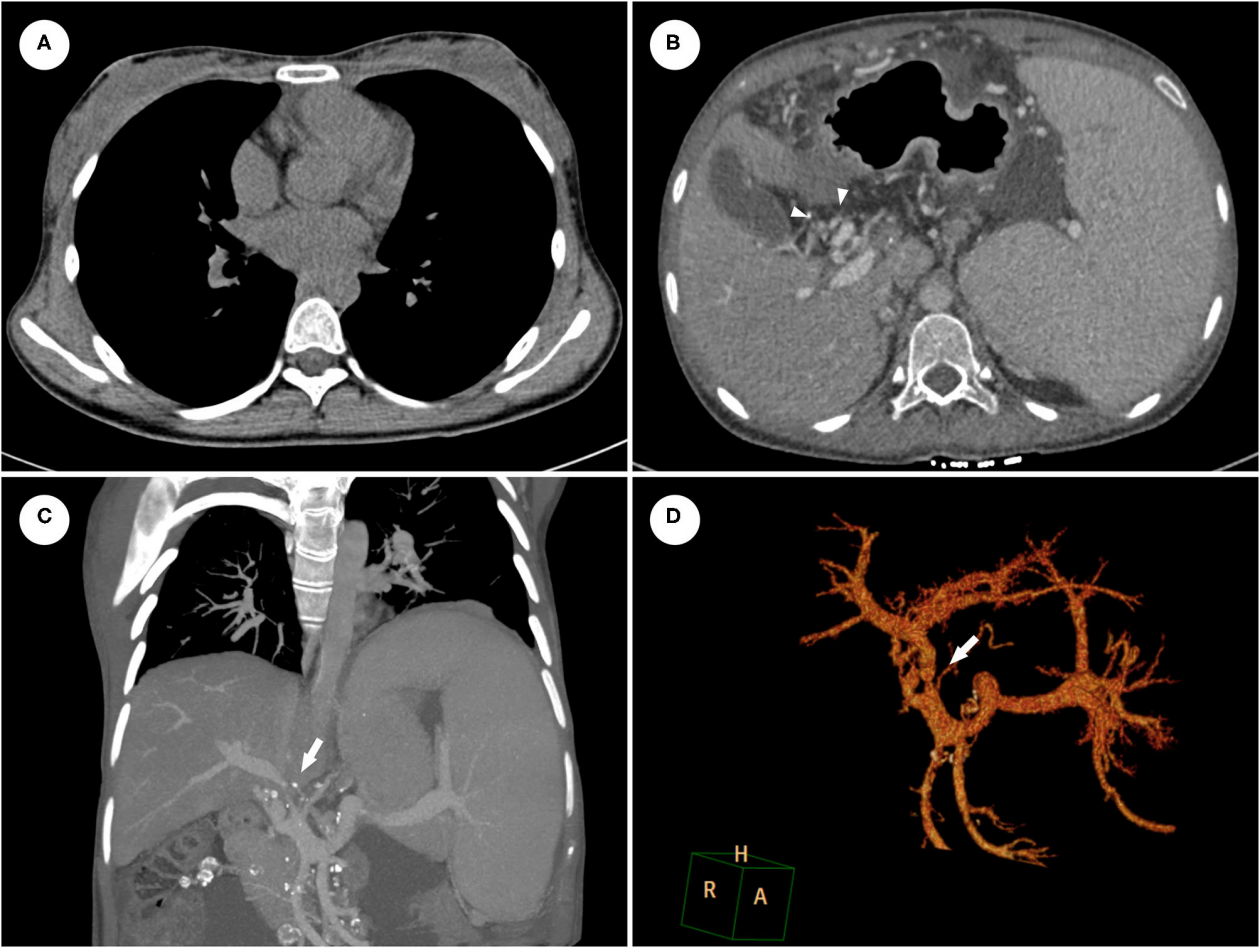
The contrast-enhanced CT revealed that pleural effusion disappeared (A) but CTPV [(B), arrowheads] was not improved after ATT. CTA with three-dimensional reconstruction showed severe stenosis of main portal vein (C,D).
